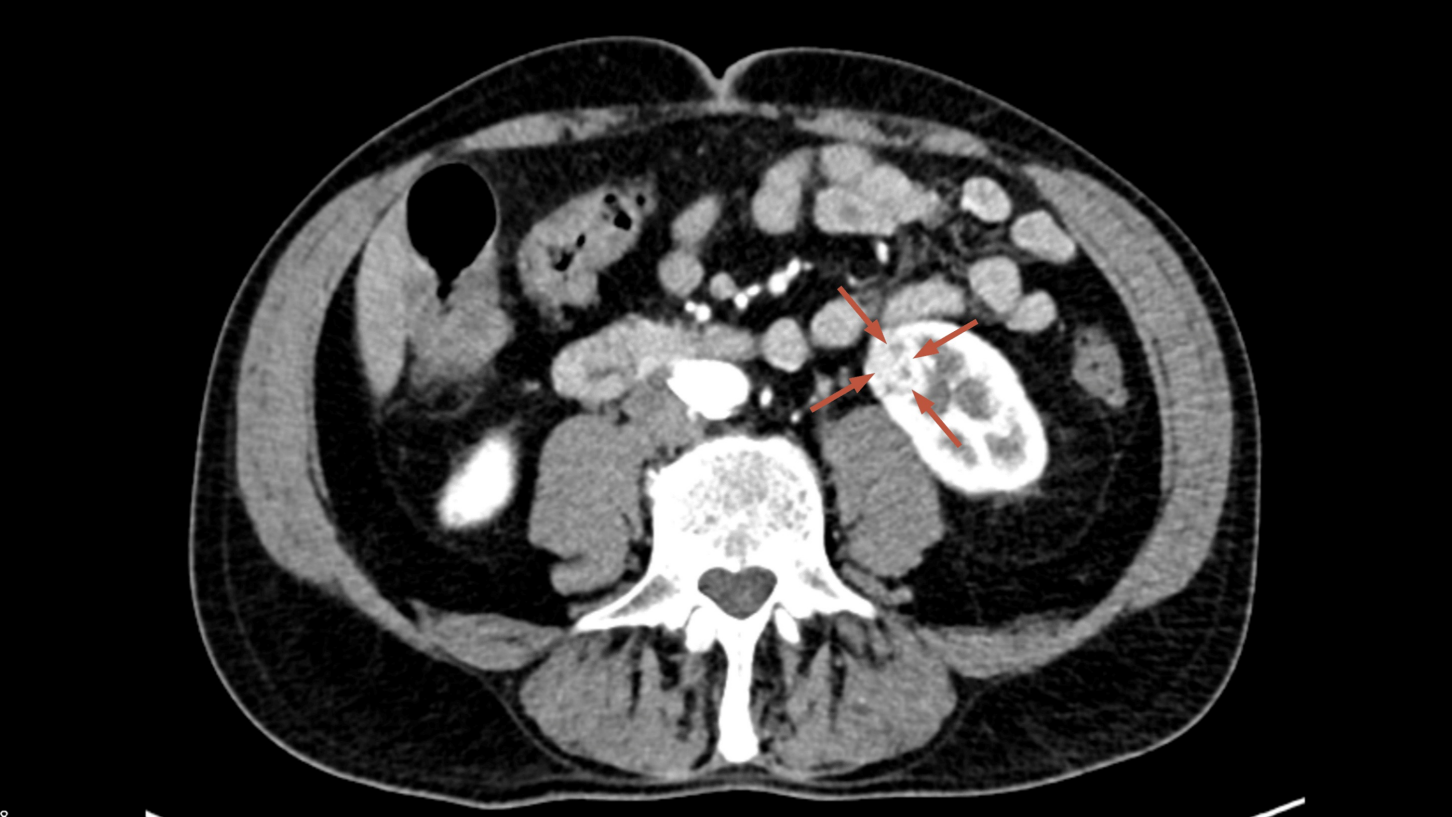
Contrast-enhanced CT of the abdomen showing a left renal mass Contrast-enhanced axial CT showing an 18 × 22 mm enhancing lesion in the mid-portion of the left kidney (arrows), containing areas of macroscopic fat and radiologically consistent with a renal cell carcinoma. There is no invasion of the renal sinus or surrounding structures. Additional simple cortical and parapelvic renal cysts are noted bilaterally without suspicious features CT: computed tomography
